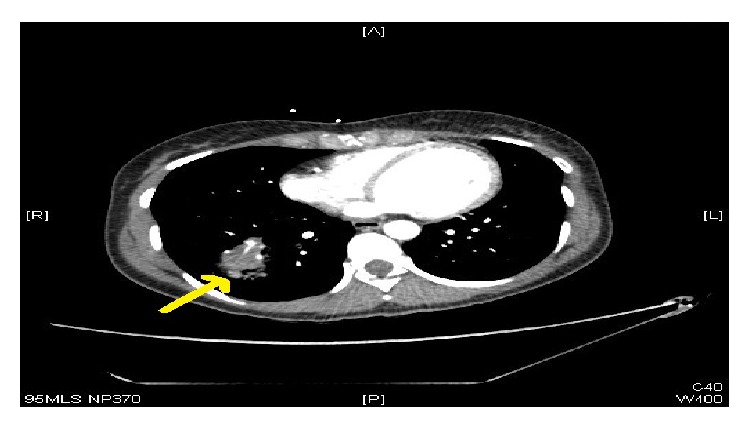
CT scan with contrast showing a right lung abscess, the most likely seeding source for patient 1's invasive muscle infection.
